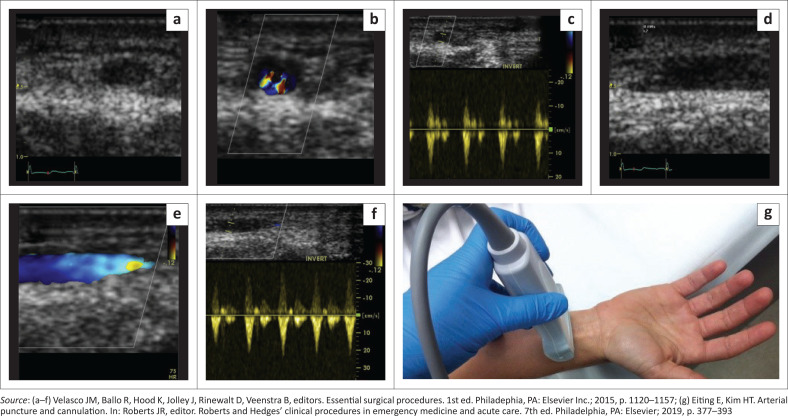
(a–g) Surface ultrasound imaging of the radial artery.
